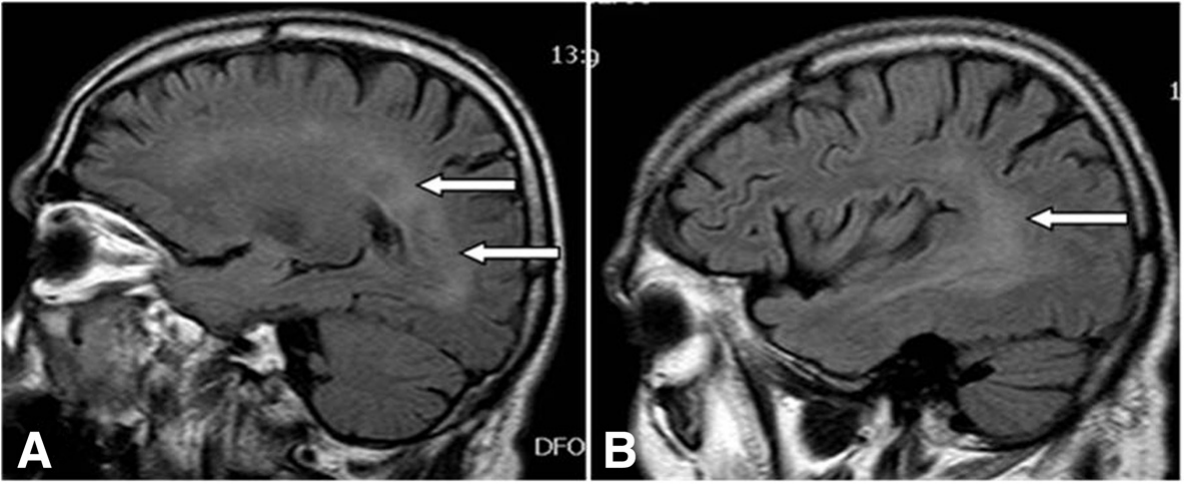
Sagittal fluid attenuated inversion recovery (FLAIR) scans (A, B) show bilateral diffuse hyperintense signal in the periventricular white matter of the parietal, occipital and temporal lobes (arrows).
